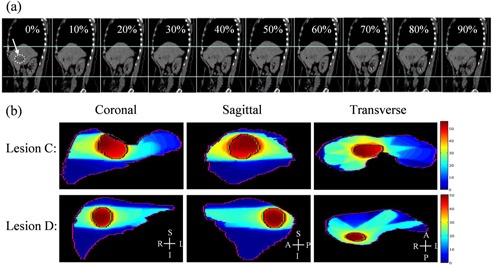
Example of sagittal planes (a) generated from the 4D CT sets for Lesion B. The arrow at 0% indicates the target region (indicated with a dotted line). The upper and lower horizontal lines of the figure are placed to guide the eye and help indicate the deformation of the liver and the right kidney. Dose distribution in liver (b): the planning target volumes (PTV) are indicated for Lesion C (the largest volume with the smallest motion) and Lesion D (the smallest volume with the largest motion) in three planes.
